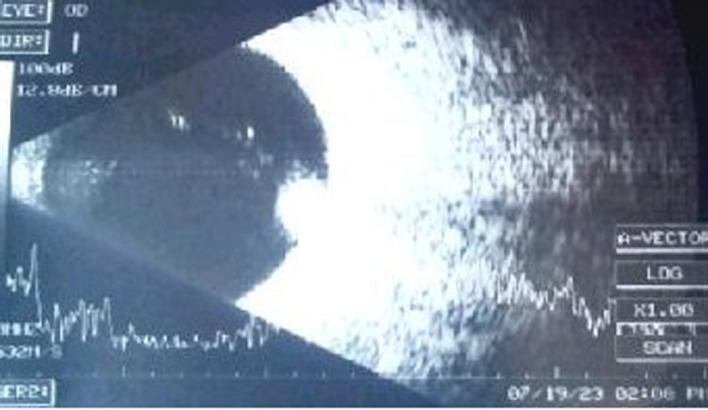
ultrasound B scan of the right eye shows a highly echogenic mass lesion protruding from the optic disc into the vitreous cavity
